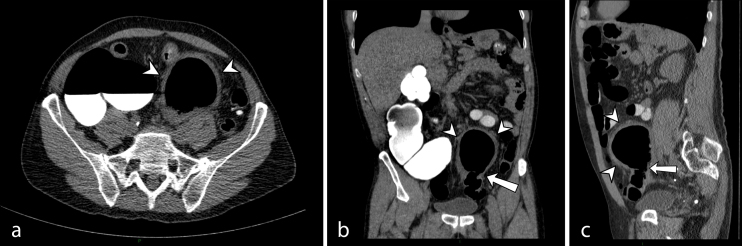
(a) Axial, (b) coronal and (c) sagittal sections of abdomen and pelvis computed tomography colonography demonstrating a large, midline cystic-appearing structure with surrounding inflammatory stranding (arrowheads). Communication to the sigmoid colon can be seen (arrow).
